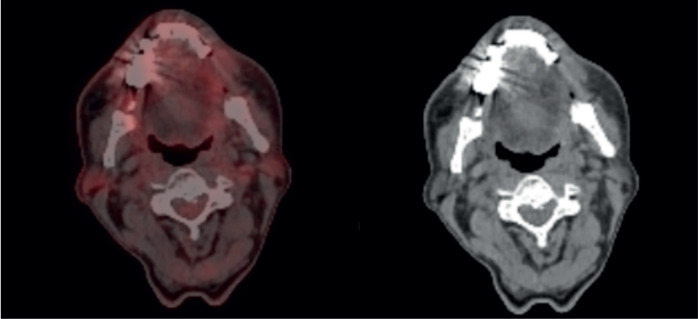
Post treatment follow-up PET CT scan, showing complete metabolic response.
